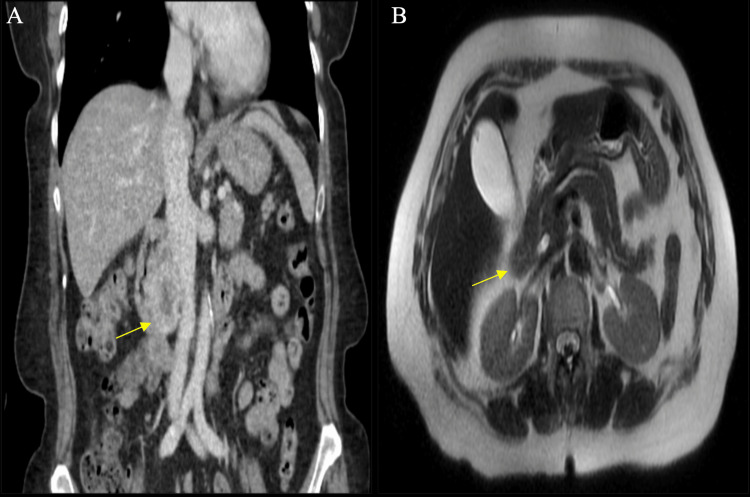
Initial CT and MRI abdominal imaging demonstrating a pancreatic mass A: CT sagittal view of mass. B: MRI demonstrating heterogenous enhancing mass within proximity of right renal vasculature and adjacent duodenum
